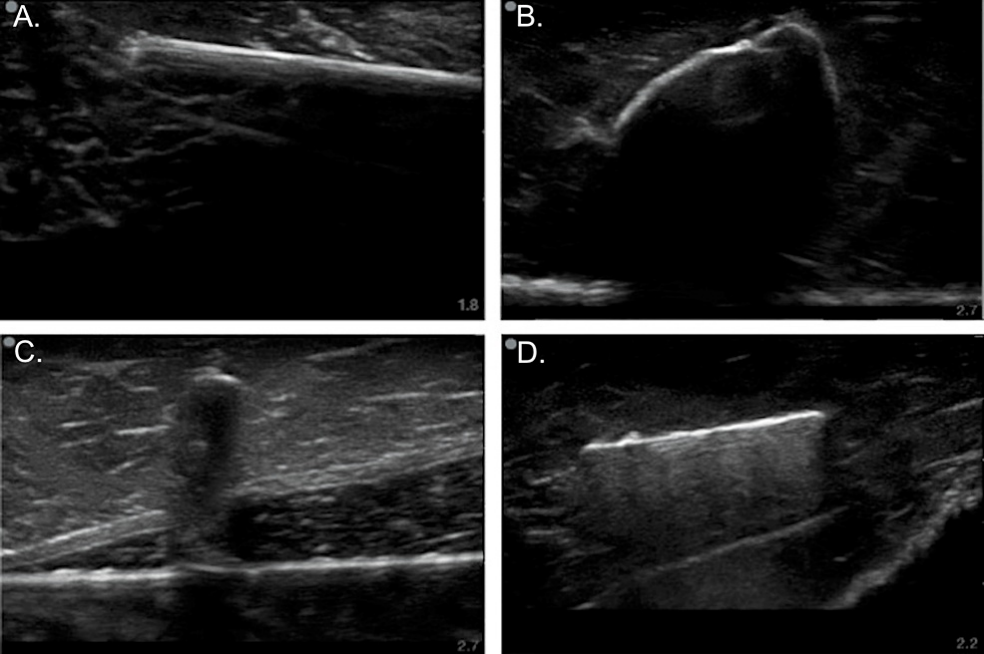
Ultrasound images of foreign bodies Images obtained using the handheld Lumify linear 12- to 4-MHz probe (Philips, Amsterdam, Netherlands)
